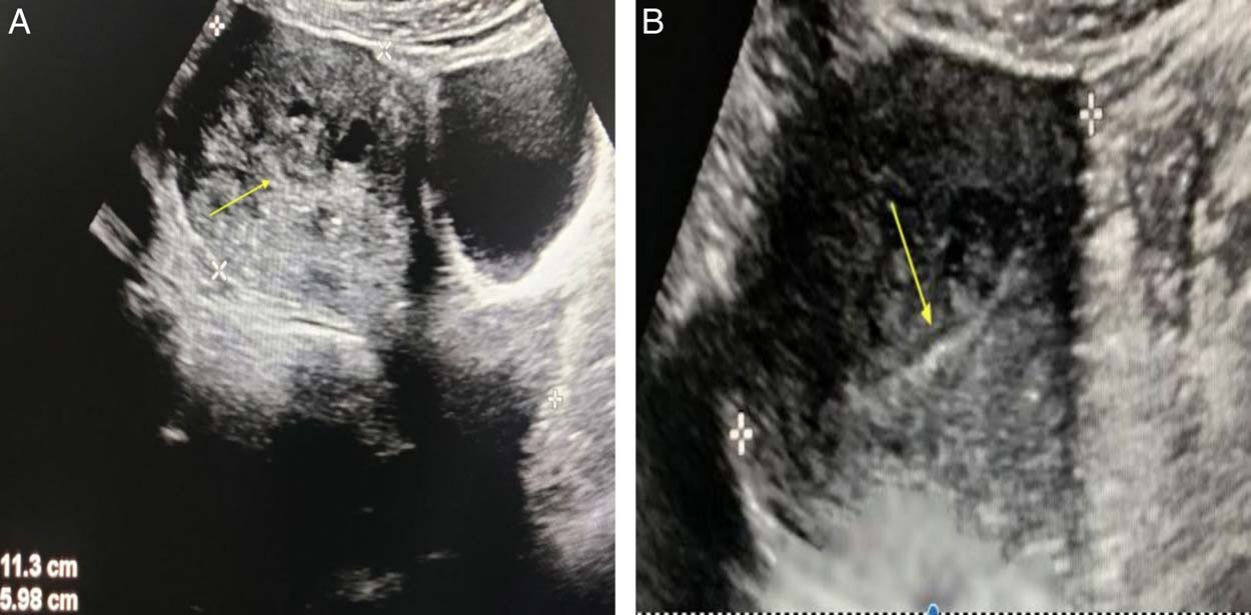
Longitudinal (A) and transverse (B) gray scale ultrasound image of the uterus showing asymmetrically thickened anterior myometrial wall with multiple anechoic areas in the myometrium. Yellow arrow pointing to the endometrium.
